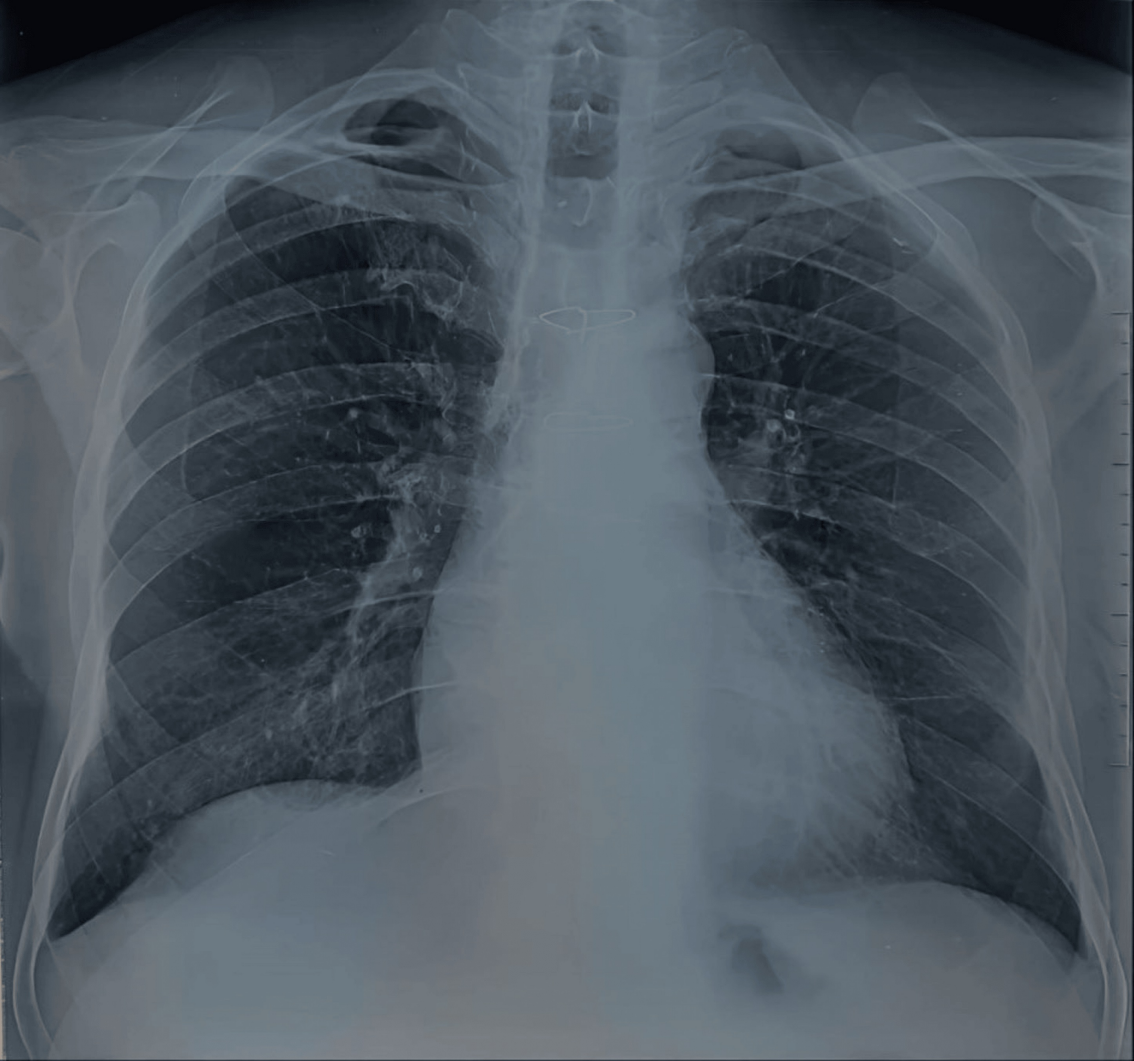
Postoperative day 7 chest X-ray showing the disappearance of the material at both the level of the innominate vein and the right ventricle, as well as the regression of the left pleural effusion
